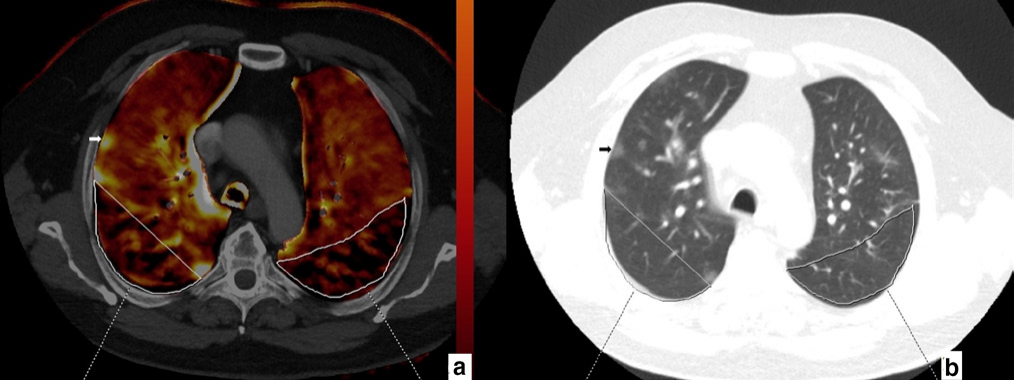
67-year-old male. Axial perfusion blood volume (a) and computed tomography (b) images. Large, heterogeneous perfusion deficit areas are seen in the posterior zones of upper lobes (a, marked areas). No GGO or consolidation is present in the perfusion deficit areas (b, marked areas). Ground glass opacification in the right upper lobe peripheral zone (b, arrow) does not reveal any perfusion deficit (a, arrow).
